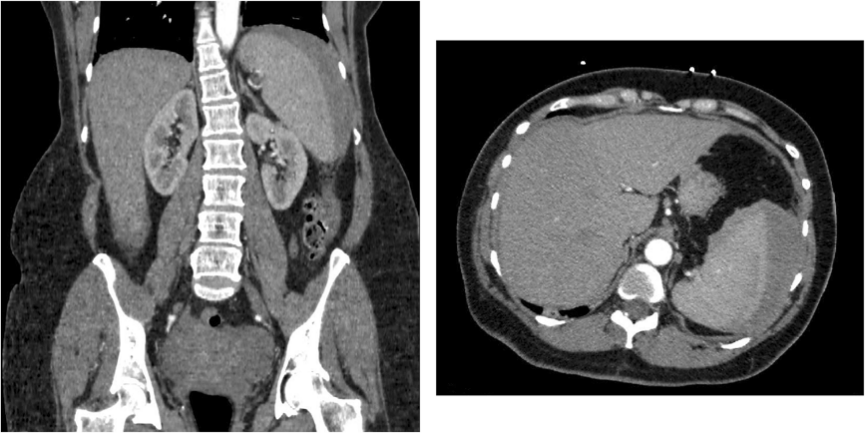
Computed tomography images of atraumatic splenic rupture. Representative sagittal (left) and axial (right) computed tomography images of the patient’s abdomen taken on the day she presented to the hospital. Grade 3 splenic injury (crescent-shaped subcapsular hematoma measuring up to 3cm in thickness along the lateral border of the spleen with lobulated regions of hemorrhage along the superior and medial border of the spleen and left subdiaphragmatic region, and linear densities in the spleen) and marked splenomegaly can be seen.
